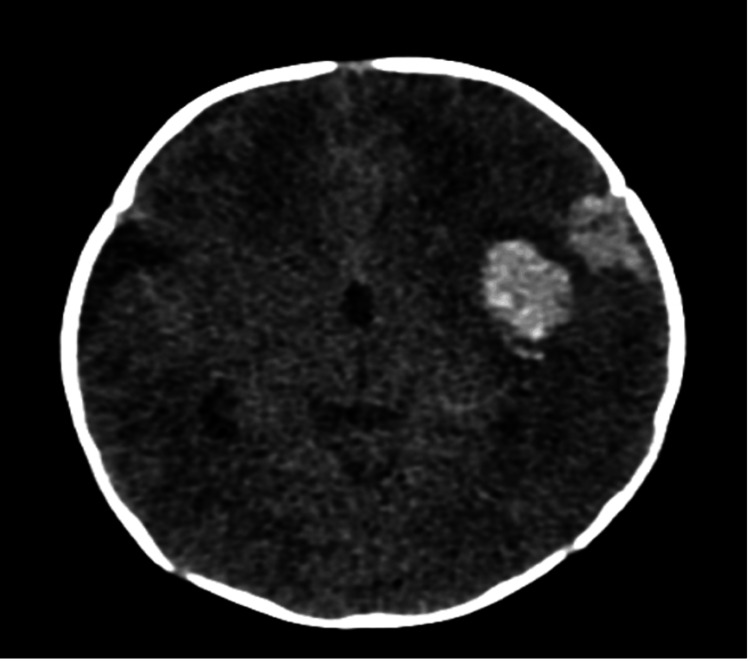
Admission brain computed tomography (CT) scan showing a voluminous intraparenchymal hemorrhage
